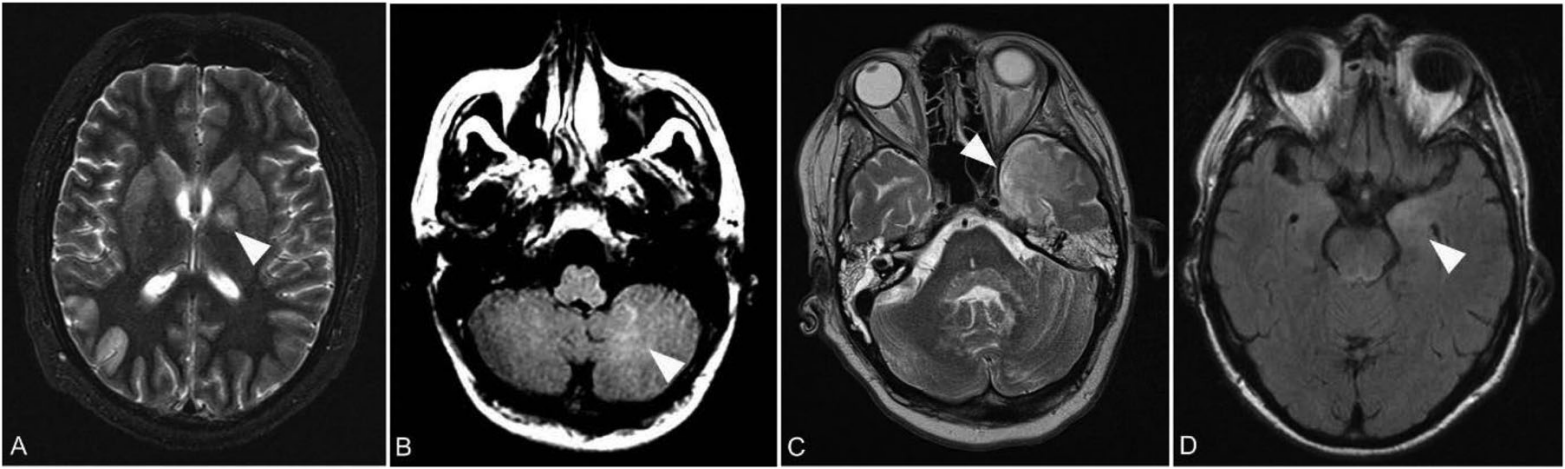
Representative asymmetric lesions. Axial T2 weighted image with left hemispheric changes of the globus pallidus (arrow) in patient B on day 39 (A). Axial FLAIR image with unilateral signal increase of cerebellar white matter (arrow) in patient D1 on day 107 (B). Axial T2 weighted image showing left hemispheric signal increase of the temporal lobe (arrow) in patient D2 on day 78 (C). Axial FLAIR image demonstrating unilateral signal increase of the left amygdala (arrow) in patient F on day 10 (D).
